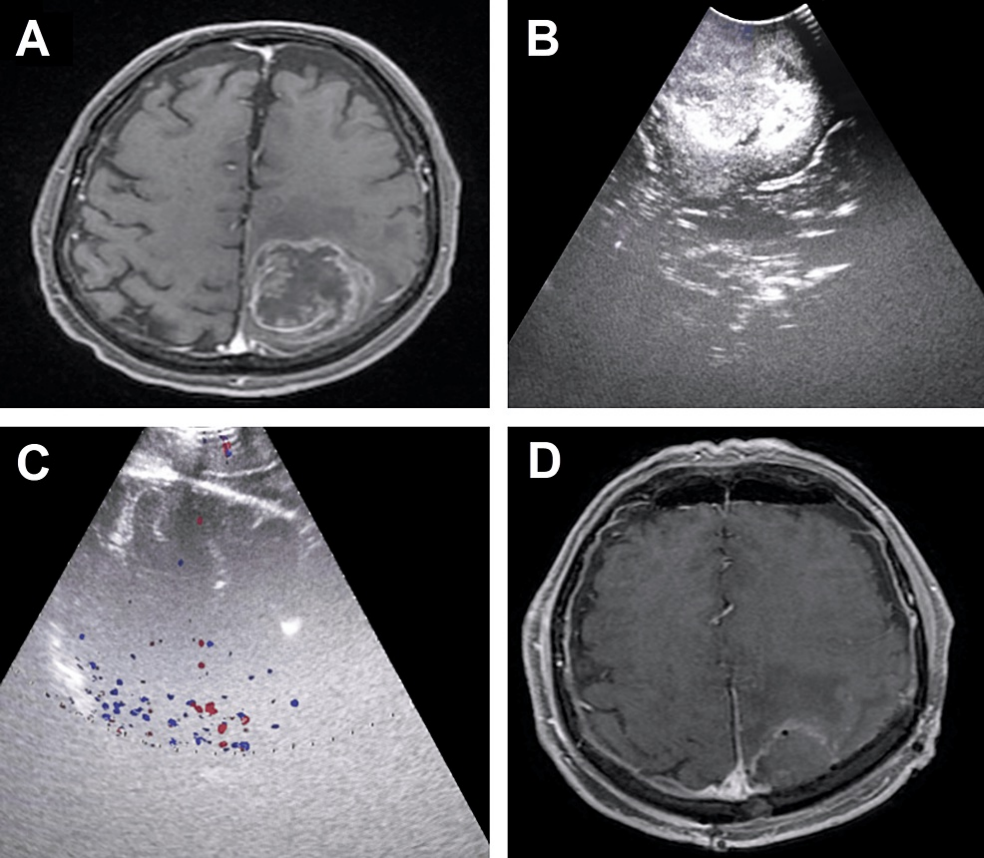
(A) Preoperative magnetic resonance imaging of a 73-year-old patient with a left parietal glioblastoma with perilesional edema. (B) Intraoperative ultrasonography image prior to dural opening where heterogeneous hyperechogenic image is observed, with hypoechogenic zones corresponding to necrosis. (C) Trans-surgical ultrasound image post-resection of the tumor where a radical resection is observed, without evidence of residual injury or bleeding. (D) Postoperative magnetic resonance imaging in which the degree of tumor resection is corroborated
